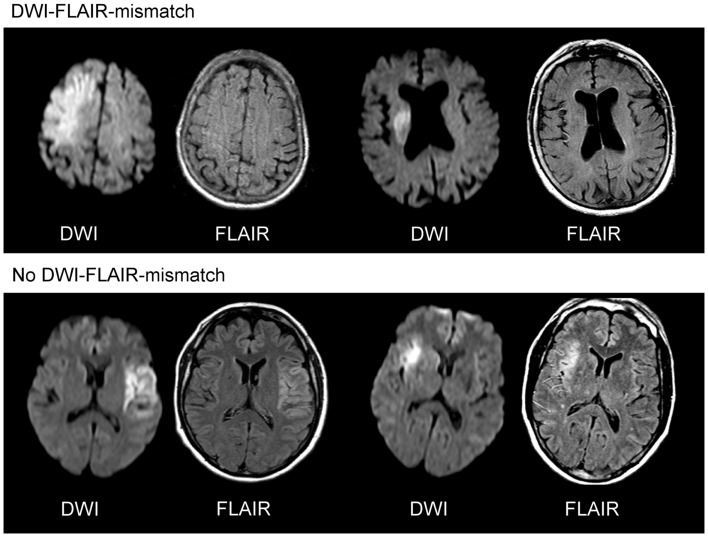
DWI-FLAIR-mismatch. The upper row gives two examples of a clearly visible acute ischemic lesion on diffusion weighted imaging (DWI), while no marked parenchymal hyperintensity is detected on fluid attenuated inversion recovery (FLAIR) images indicating DWI-FLAIR-mismatch. In the lower row, a clear hyperintensity can be seen on FLAIR images in the area of the acute DWI lesion (no DWI-FLAIR-mismatch).
